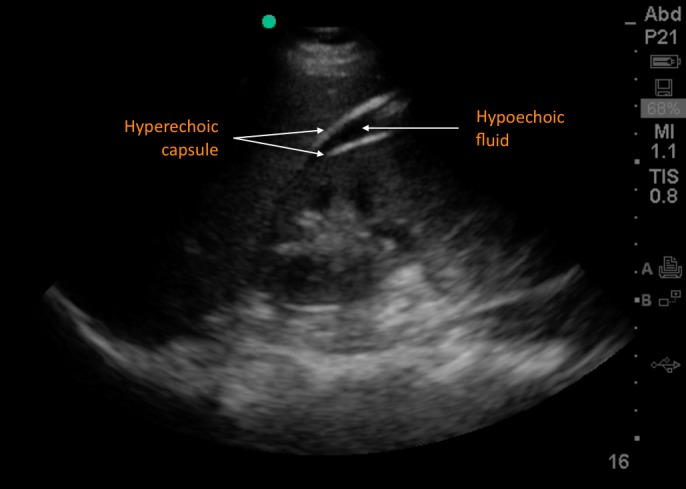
Point-of-care ultrasound visualization of the renal hematoma Right upper quadrant view demonstrates hypoechoic and heterogenous fluid between the liver and right kidney, bordered by hyperechoic capsular tissue, consistent with a subcapsular perirenal hematoma.
